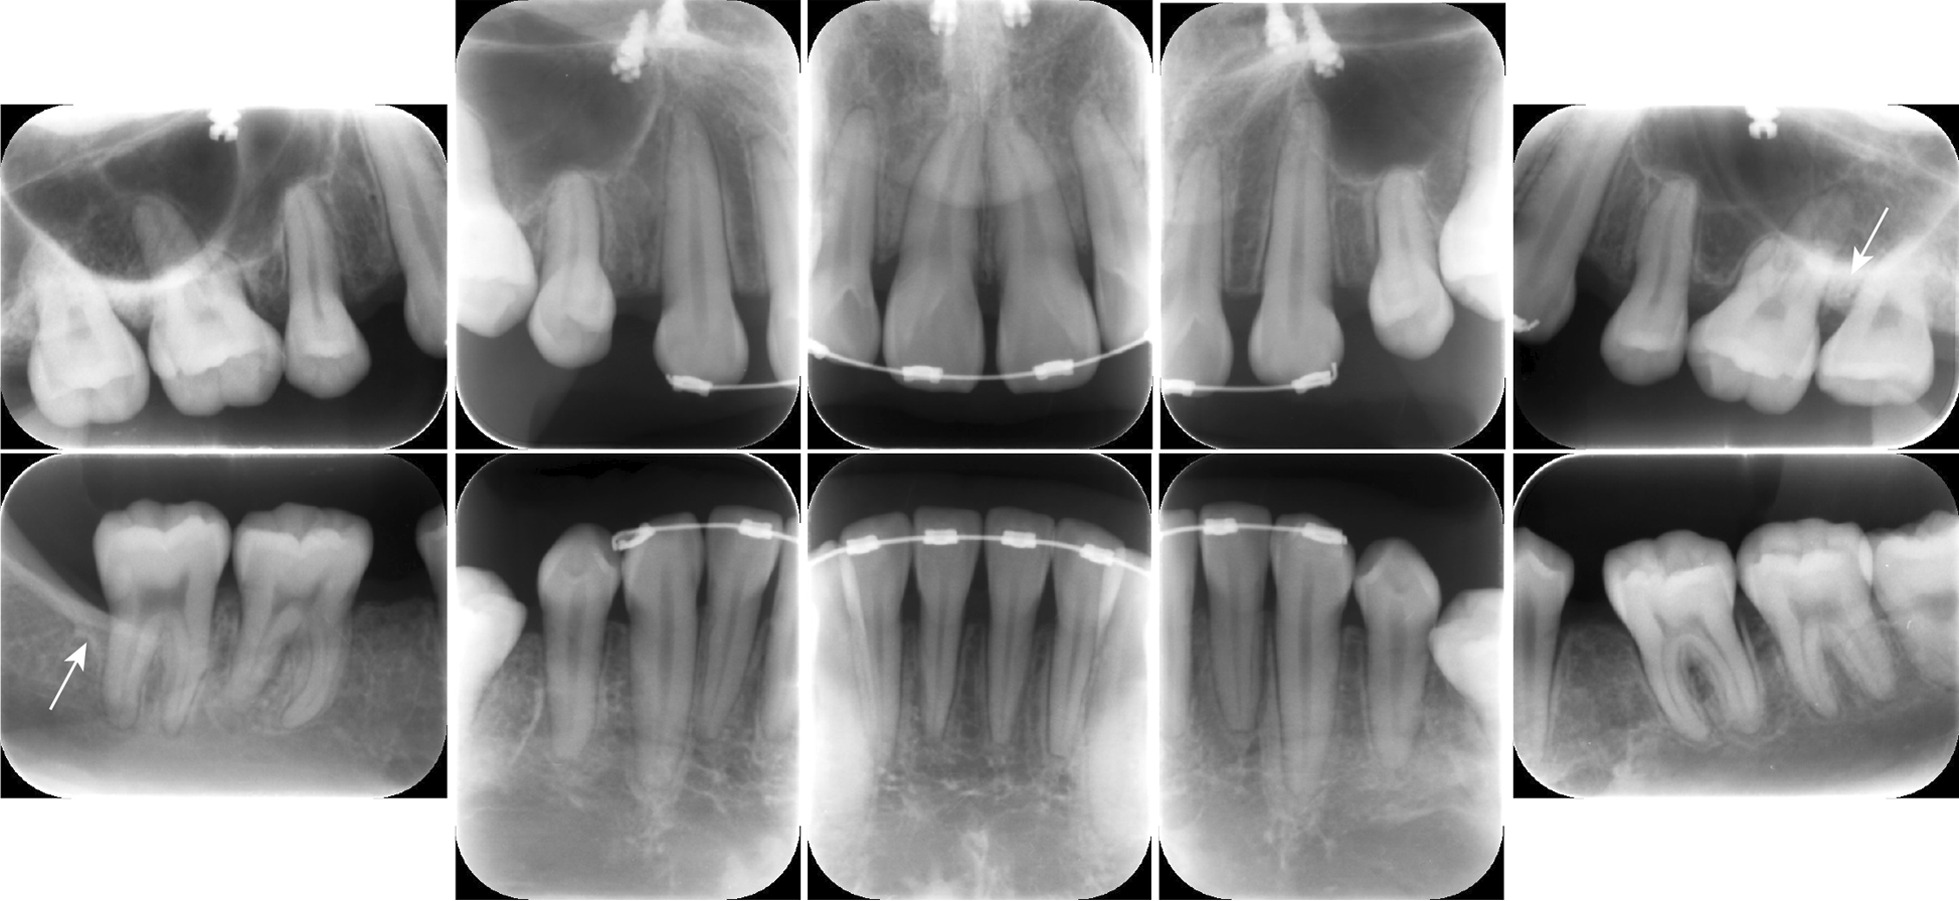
Intraoral periapical radiographs after periodontal treatment. No evidence of bone resorption progression is observed. Alveolar bone deposition can be observed on the distal aspects of the maxillary left second molar and mandibular right second molar (white arrows), thus, indicating that bone grafting is not required
